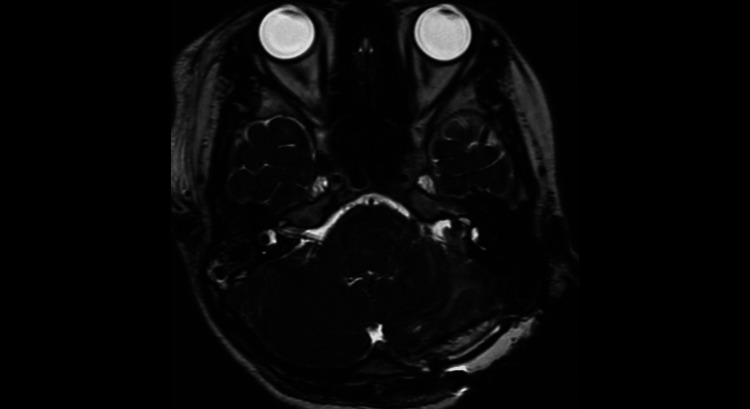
Immediately after reoperation, MRI T2-weighted image All tumors within the internal auditory canal had been completely removed.
